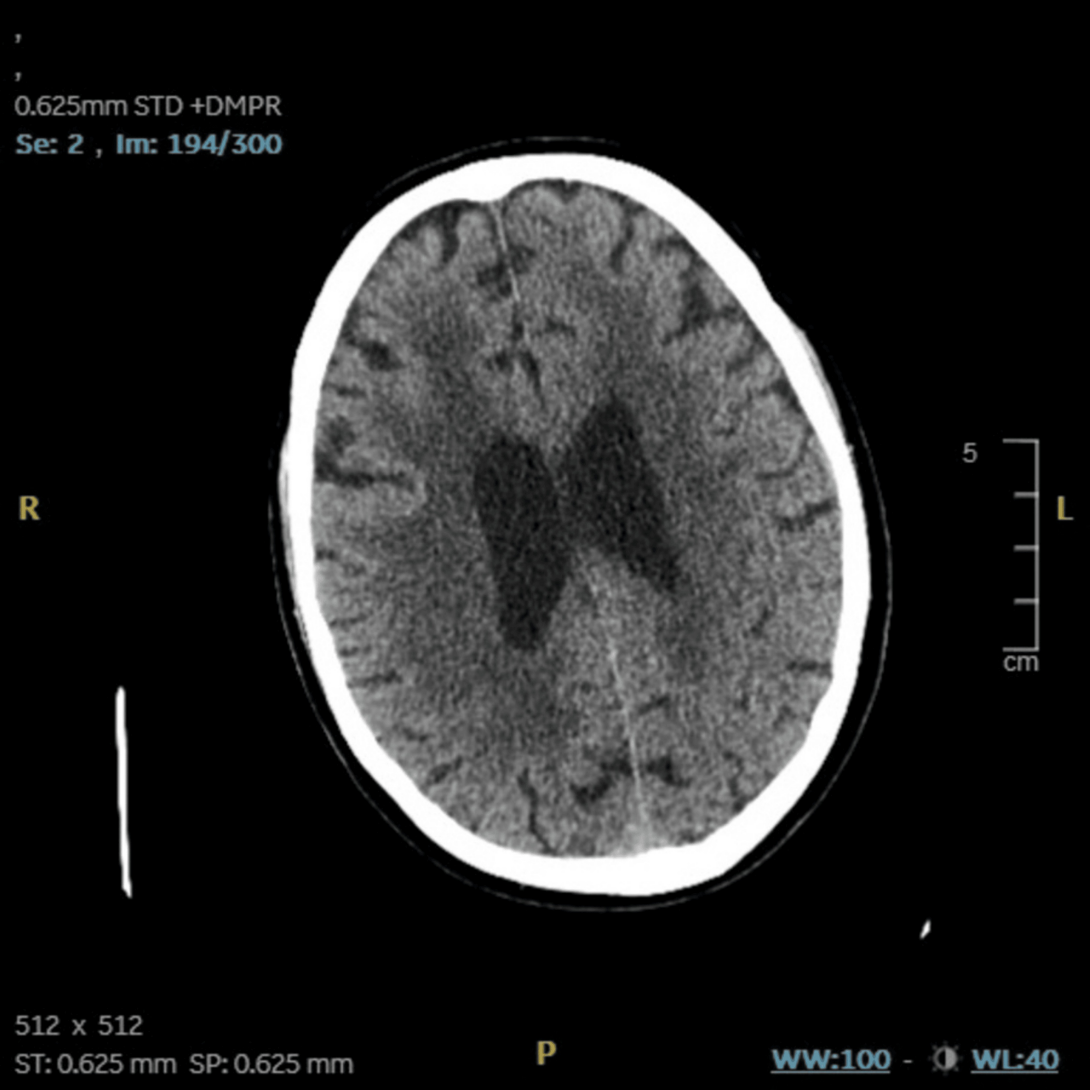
CT head - image 1 The image shows patchy areas of hypoattenuation in the cerebral white matter, consistent with moderate small vessel disease CT: computed tomography
